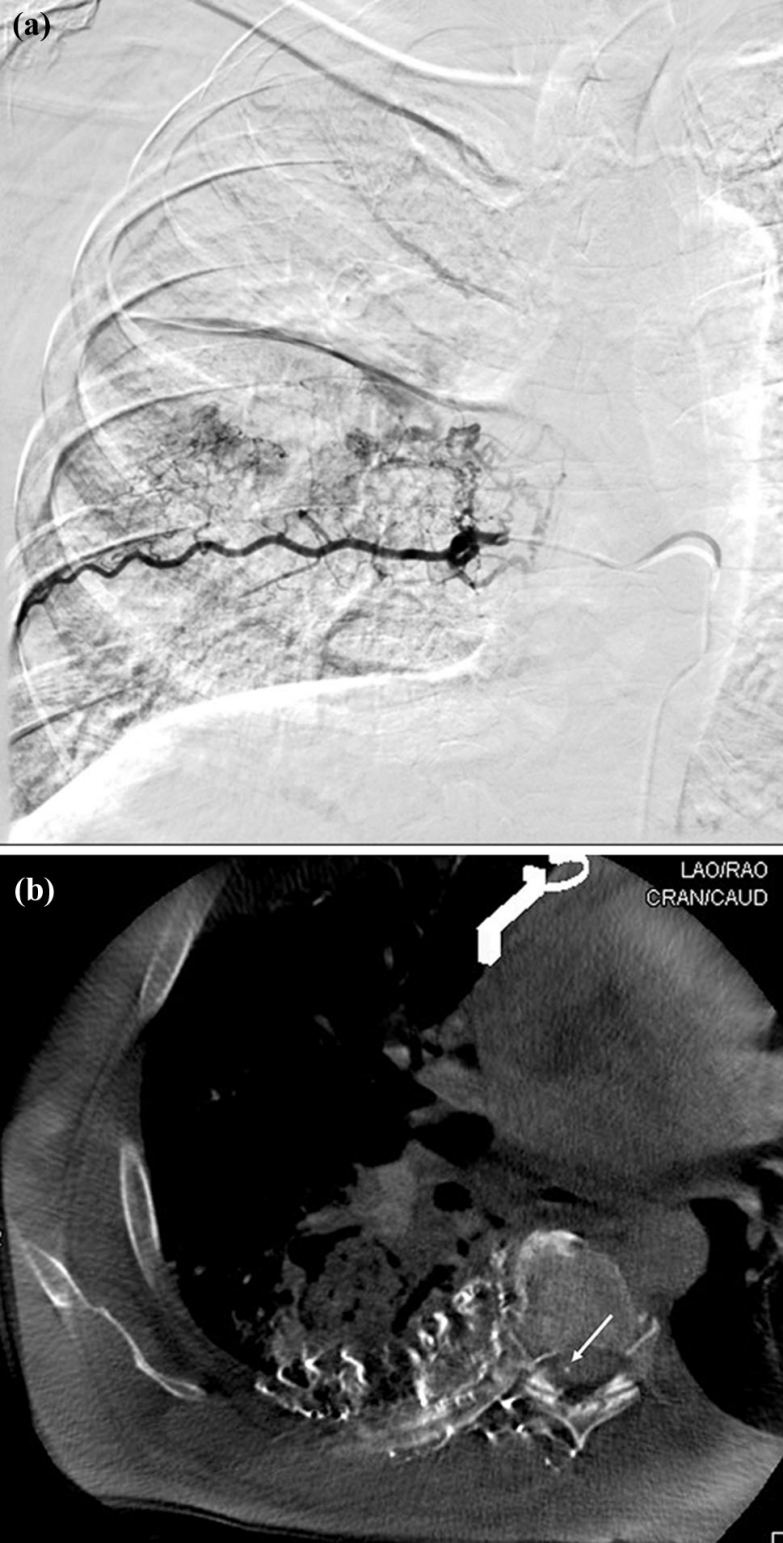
62-y/o male with history of tuberculosis and aspergilloma, who presented with hemoptysis. a Coronal DSA interrogation of the right T7 intercostal artery demonstrates abnormal lung opacification without clear evidence of a spinal artery. b CBCT at this region demonstrating arterial perfusion to the anterior spinal artery (white arrow), which was occult on DSA. This prompted disengagement of the corresponding vessel and prevented a potential non-target embolization
